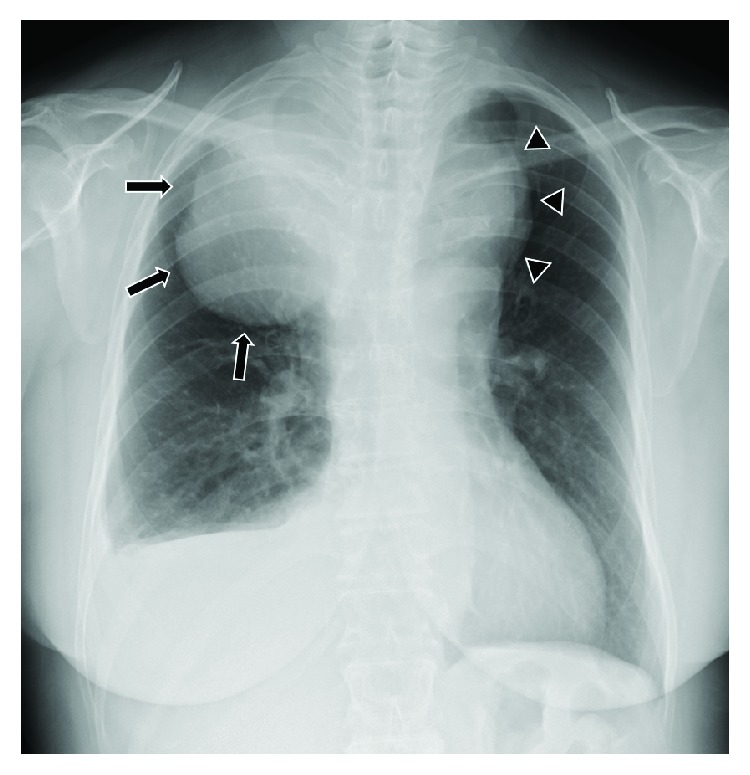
Chest X-ray findings. The chest X-ray showed a tumor shadow (arrow) in the upper-middle field of the right lung with pleural effusion and a tumor shadow (arrowhead) in the upper field of the left lung.
